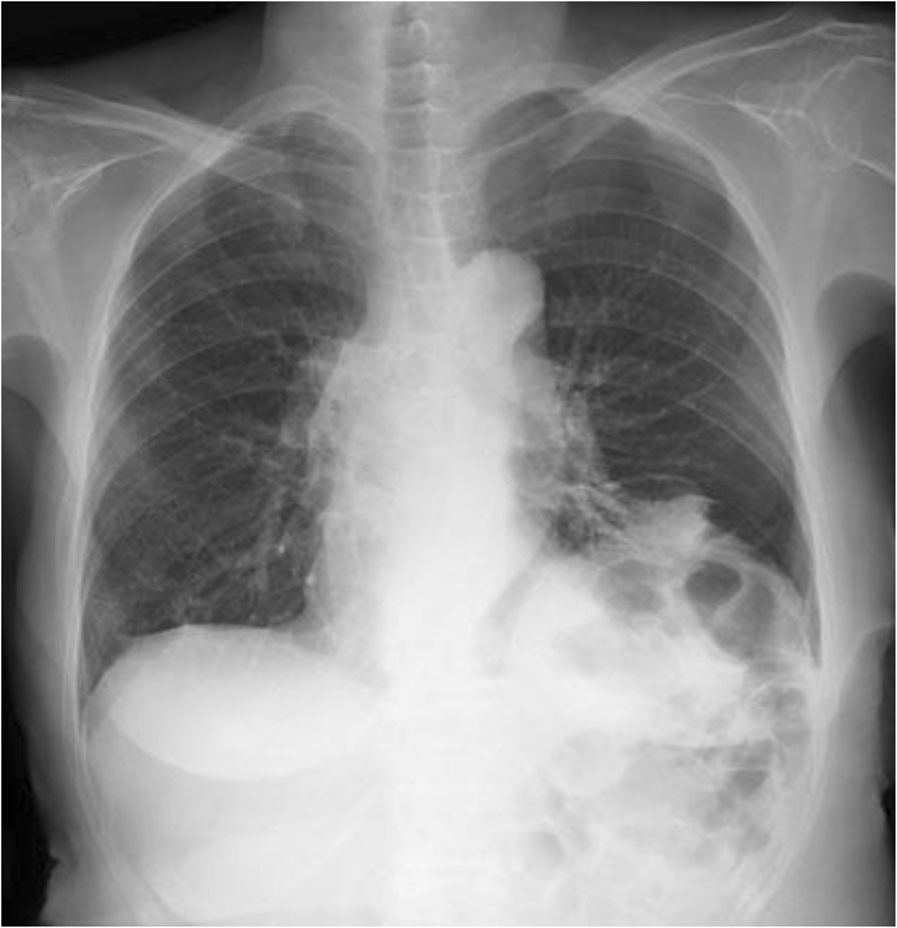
Chest X-ray after diaphragm plication. The improvement in the left lung expansion after plication
